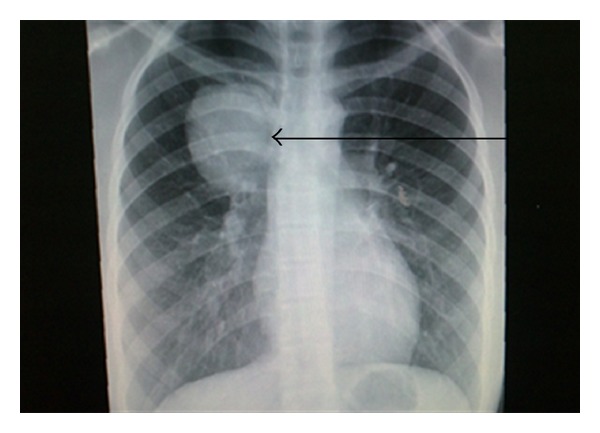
Posteroanterior radiograph of chest showing (arrow) right-sided circumscribed mass in the upper lobe close to the hilum.
